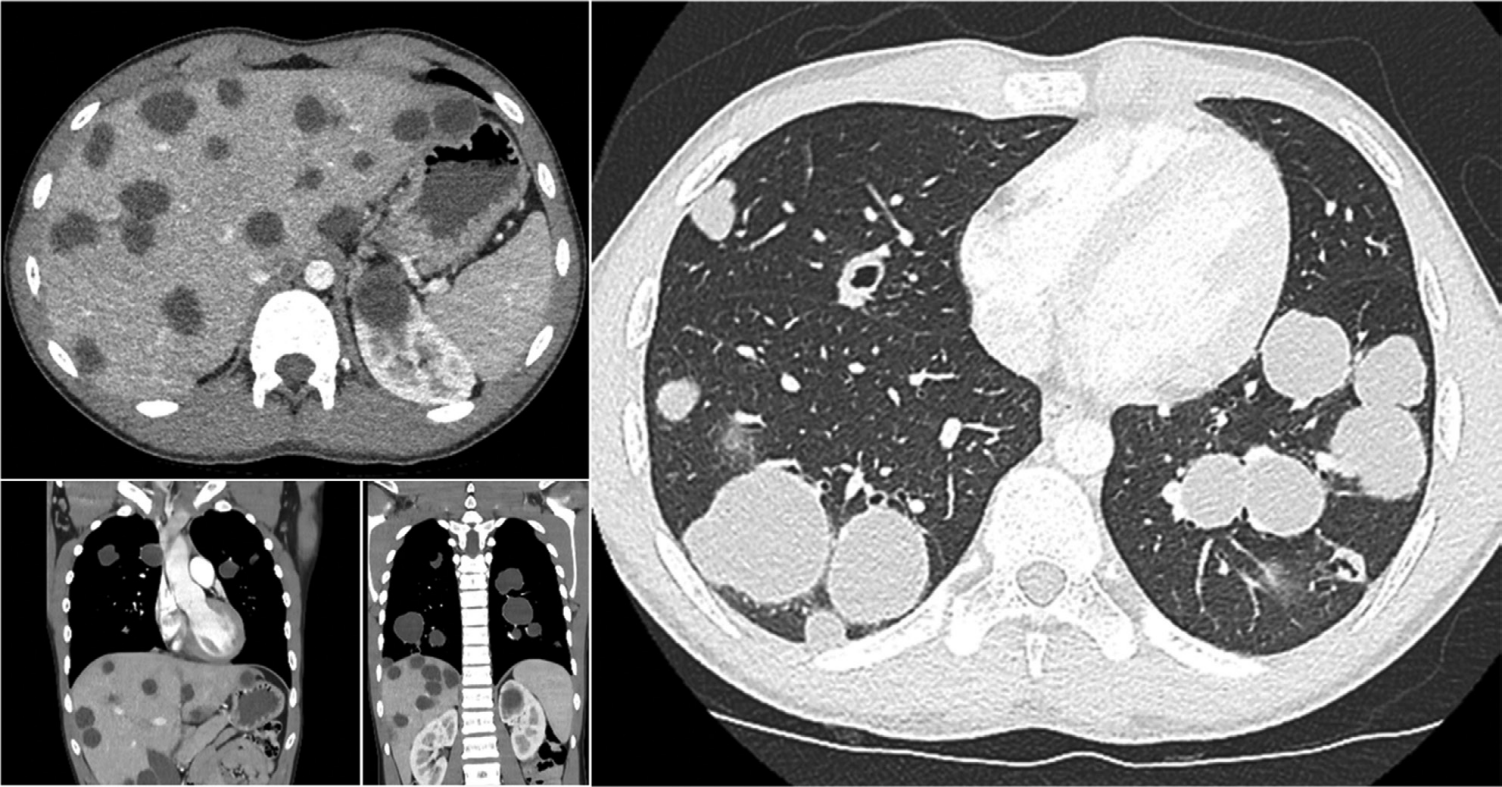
Axial CT images of the abdomen, coronal, and axial CT images of the thorax reveal plenty disseminated cystic lesions in the lungs and liver.
